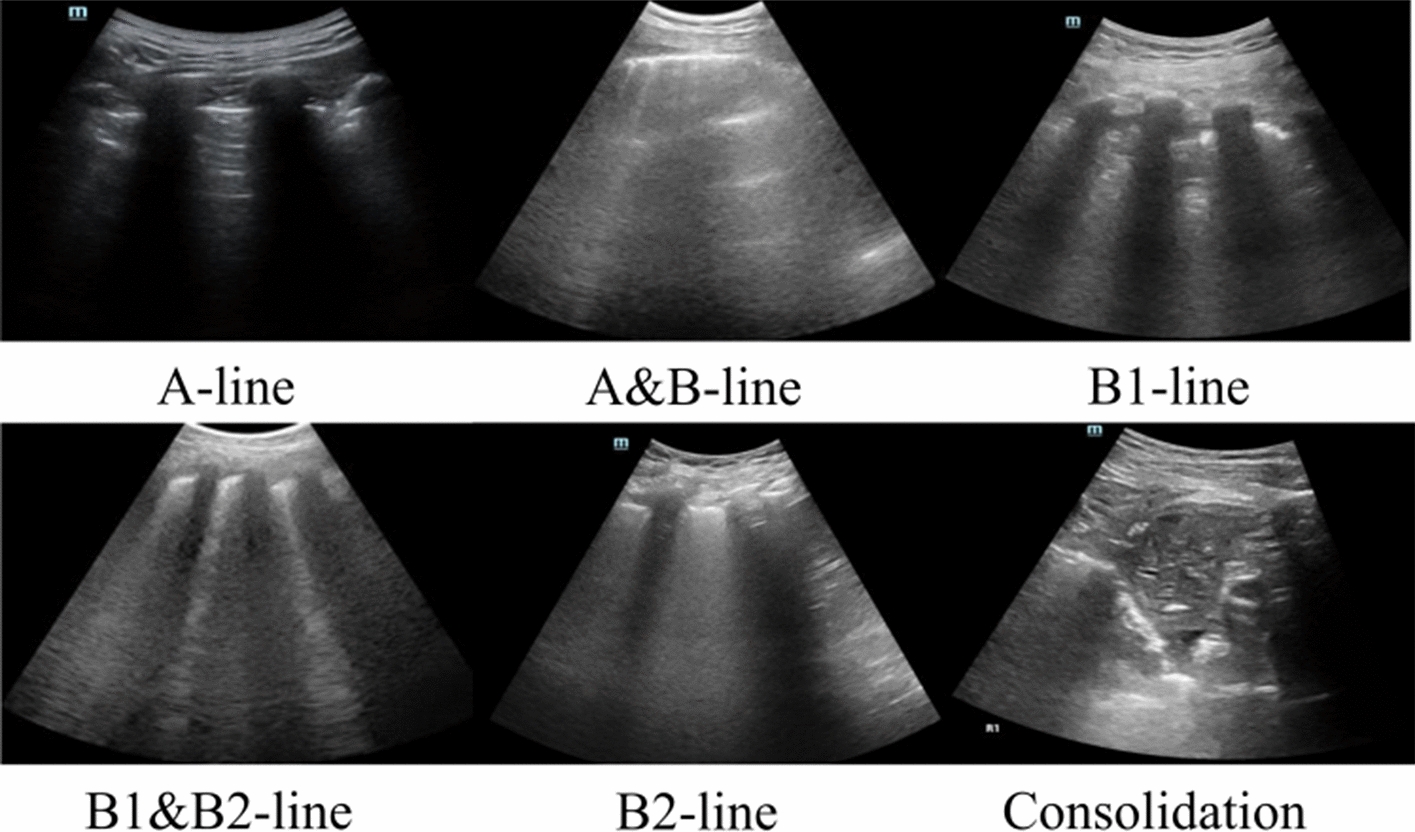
Different ultrasound sonograms in lung examination
